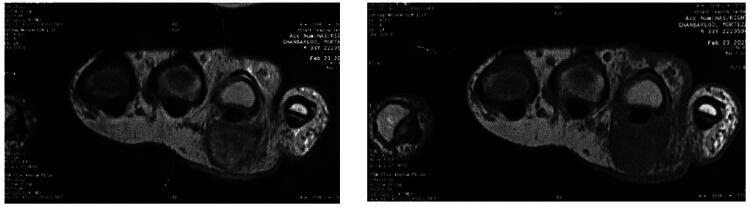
MRI scans of the right hand in axial planes showing a well-defined mass with low signal intensity on T1-weighted image (right side) and high signal intensity on T2-weighted image (left side), located on the palmar aspect of the fourth finger and surrounding the flexor tendon.
